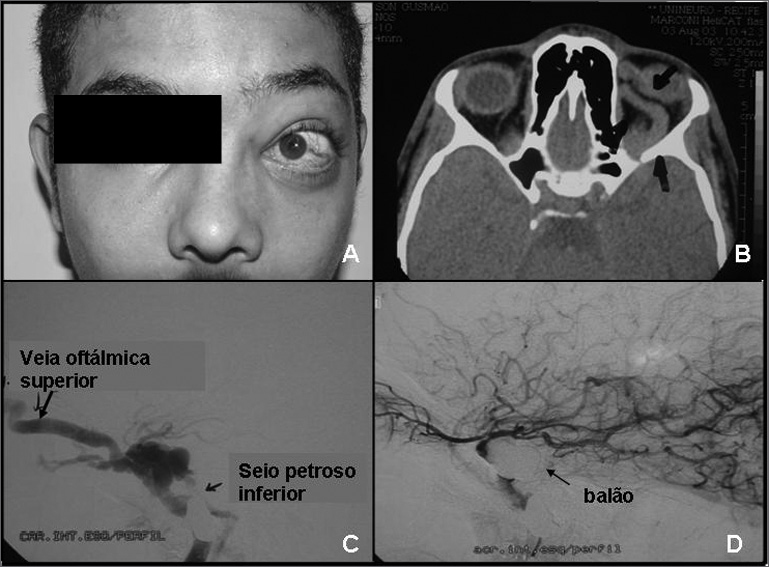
(A-D) - A - Proptosis; B - CT scan reveals much dilated superior ophthlalmic vein; C - brain digital angiography; D - carotid-cavernous fistula balloon embolization.
